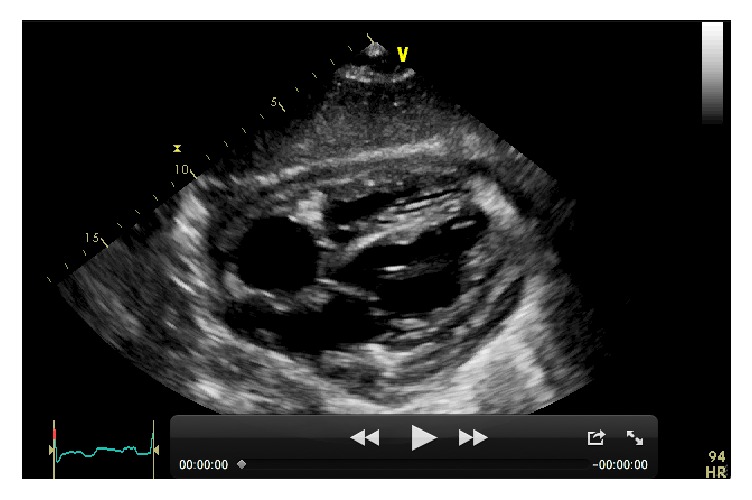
Transthoracic echocardiography.* Constrictive pericarditis. Pericardial thickening with a large amount of fibrin totally encasing the heart.
